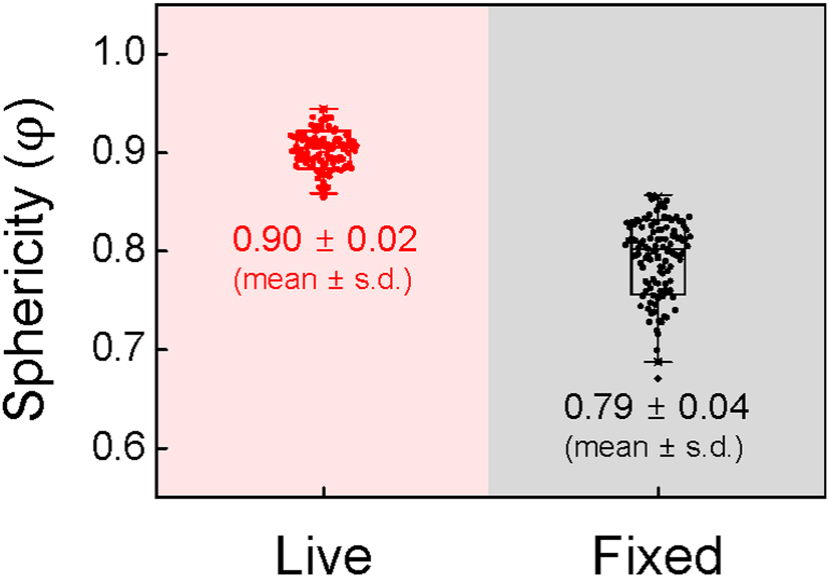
Sphericity of 120 alveoli in the live lungs and 120 alveoli in the fixed lungs. $$\mathrm{\varphi }$$ is the sphericity of individual alveoli ($$\mathrm{\varphi }$$ of the sphere = 1). $$\mathrm{\varphi }$$ = 0.90 ± 0.02 (mean ± s.d.) for the live lungs and $$\mathrm{\varphi }$$ = 0.79 ± 0.04 (mean ± s.d.) for the fixed lungs were taken from x-ray microtomography (*P*-value = 2.8 × 10^–67^). Every single measurement is not from the same alveolus.
